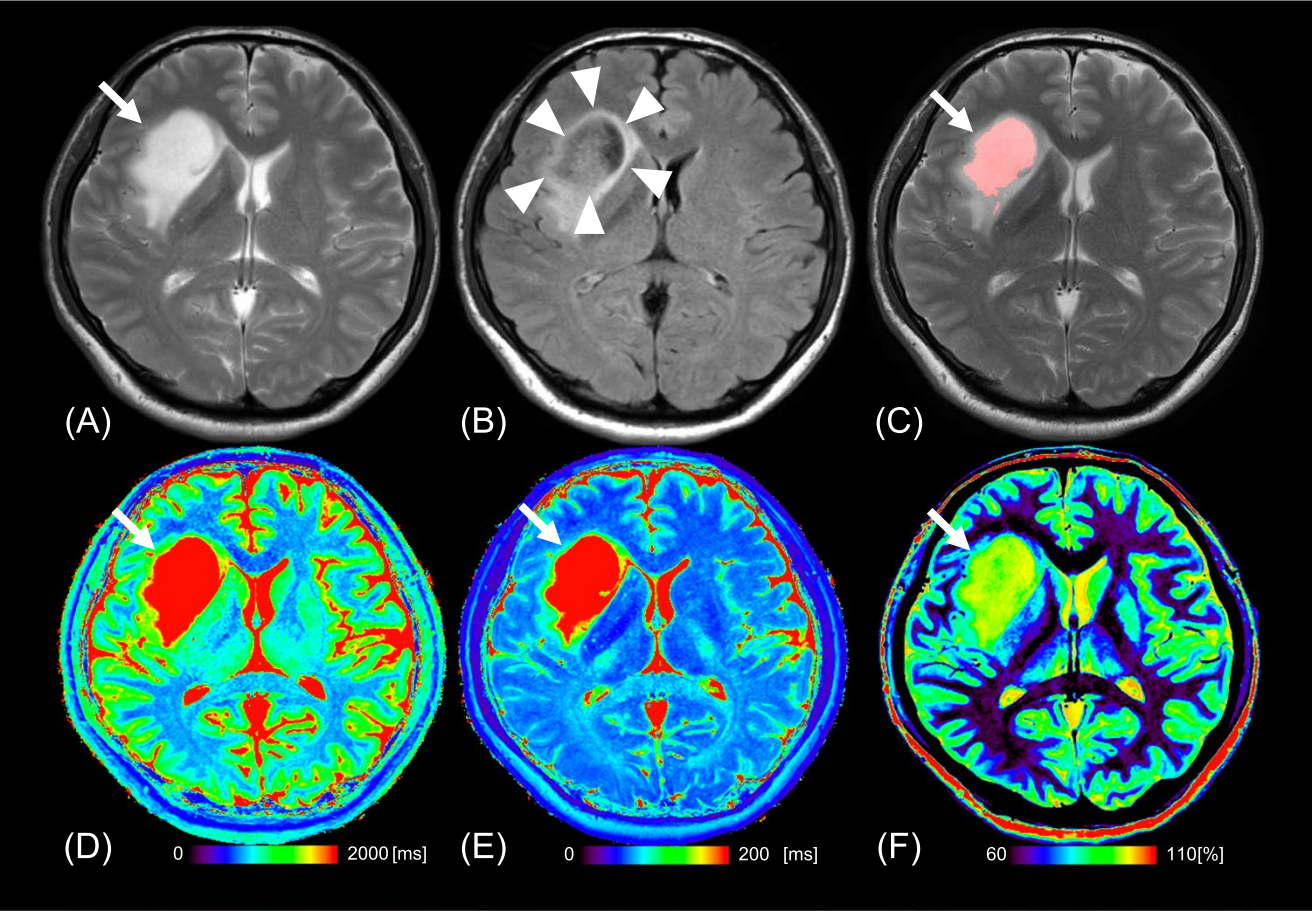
Images from a 39-year-old man with astrocytoma, IDH-mutant (WHO Grade 2). a T2WI shows a homogeneous T2-prolonged mass in the right insula (arrow). b FLAIR shows partial signal suppression, indicating a T2-FLAIR mismatch sign (arrowheads). c Our artificial intelligence correctly detects this T2-FLAIR mismatch lesion (arrow). T1 (d), T2 (e), and relaxation time and proton density (f) maps derived from SyMRI show T1 (2891 ms*) and T2 (375 ms*) relaxation time prolongations and increased PD (96.1%*) (arrows) in the tumor Asterisks (*) beside the values in the caption indicates that each value is expressed as the mean
